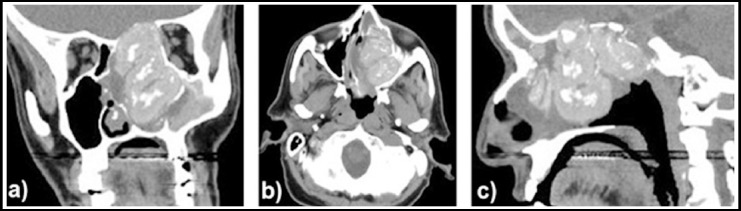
Computed tomography images of adult male patient revealed multiple polyps in the left paranasal sinuses a) coronal section, b) axial section, and c) sagittal section.
